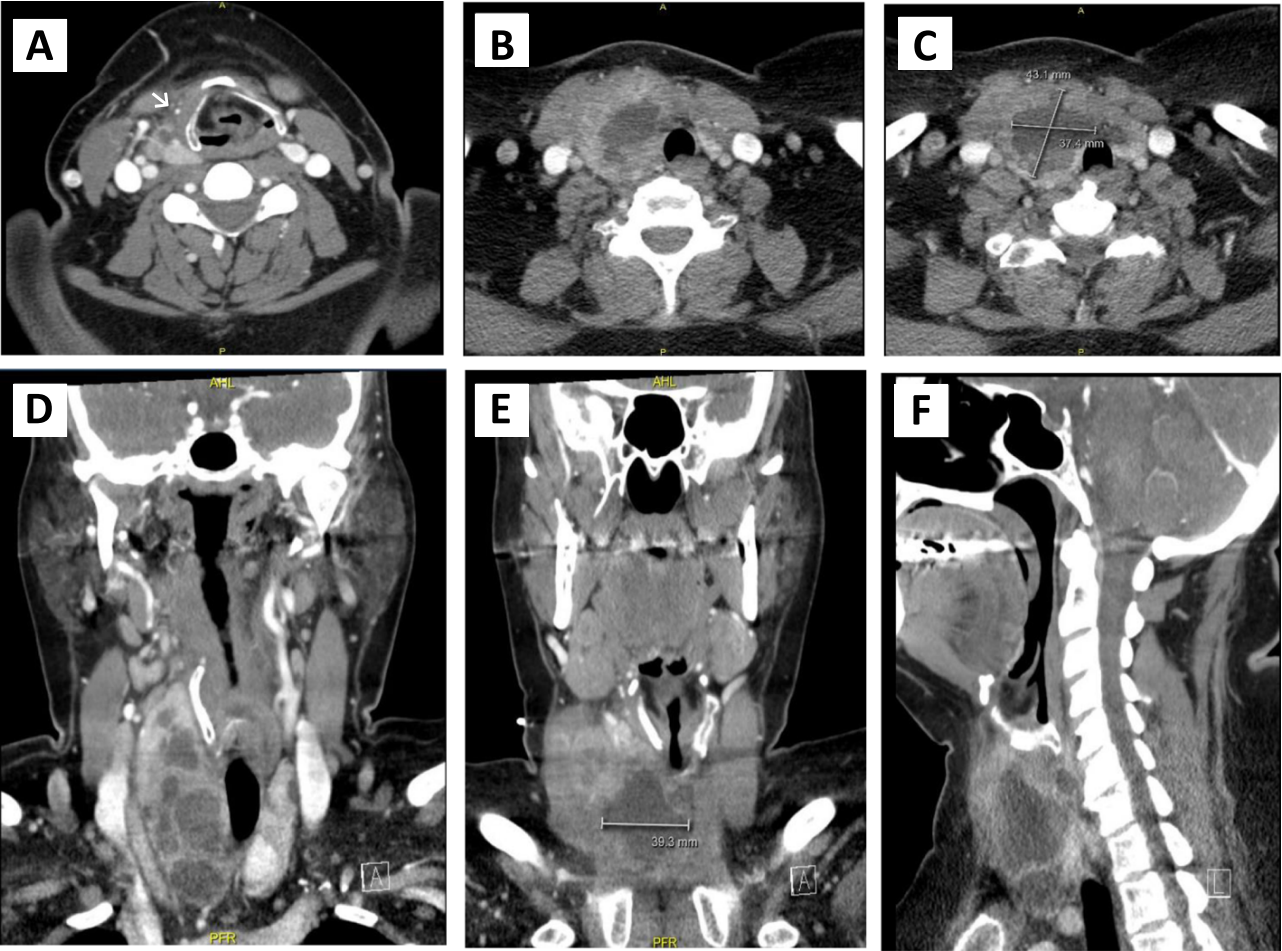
An enhanced CT scan of the neck after antibiotic therapy. a-c showing the axial views from superior to inferior, d-e showing the coronal views from anterior to posterior and f showing the sagittal view. The images show a loculated cystic lesion with internal septations in the mid lower lobe of the right thyroid which measures 3.7 × 4.3 × 5 cm in size. However, it has a progressively thickened heterogeneously enhancing wall. The white arrow on figure a pointing the right infrahyoid muscle that is slightly thickened with adjacent inflammatory stranding
